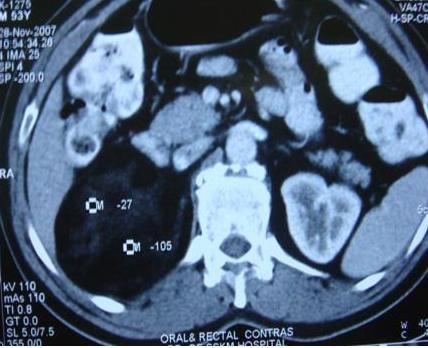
Axial section CT scan of the right sided adrenal myelolipoma.
